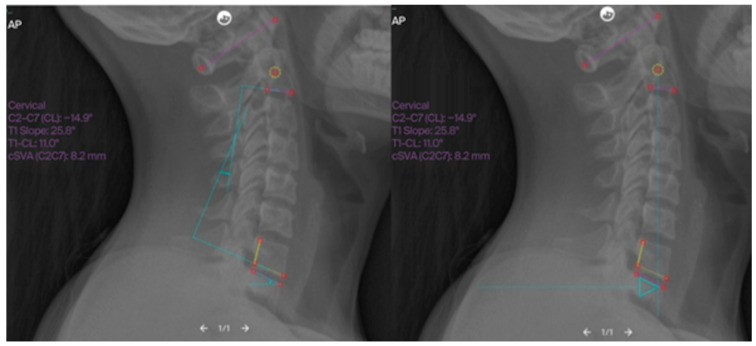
Examples of CL, T1S, and cSVA measurements using Surgimap^®^. (Left): Lateral cervical radiograph with measurements of cervical lordosis and T1 slope. (Right): Lateral cervical radiograph with measurement of cervical sagittal vertical axis. Source: Surgimap^®^ software, version 2.3.2.1 (Nemaris Inc., New York, NY, USA).
